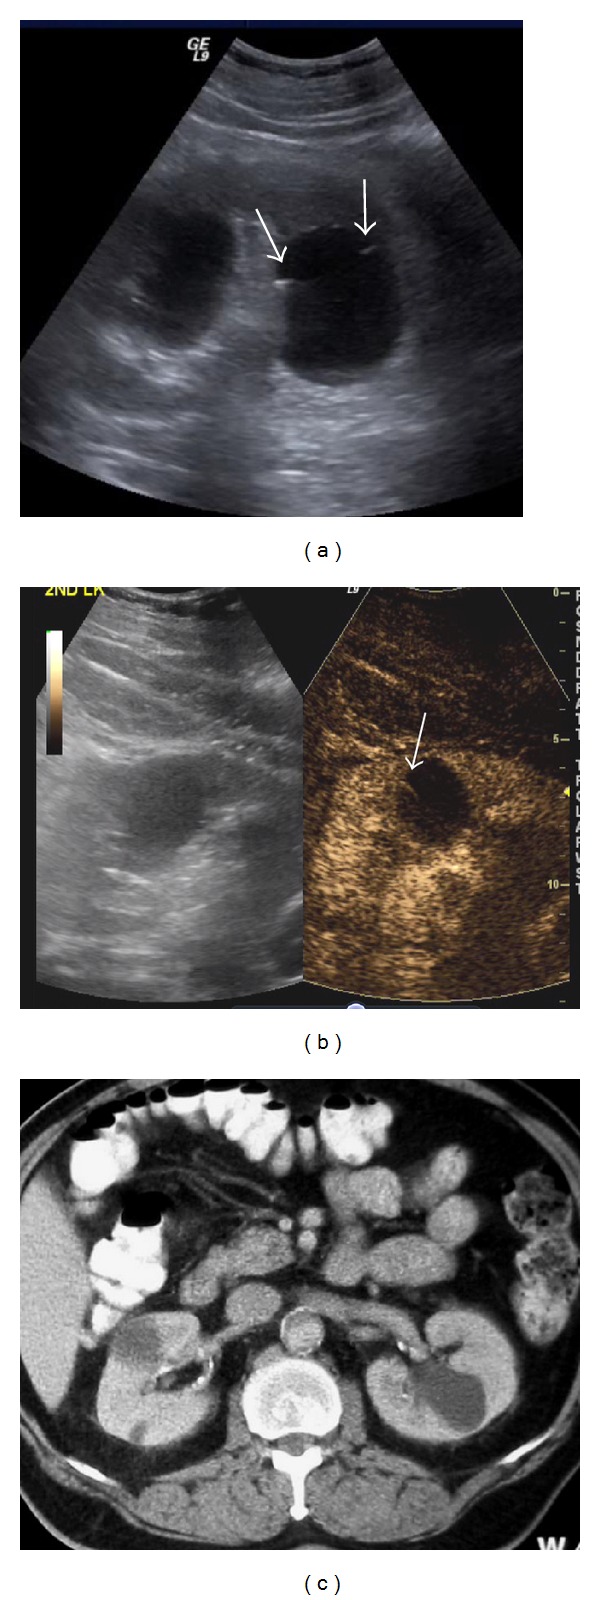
Bosniak IIF cyst: two cysts are present in the left kidney on B-mode US (a). The larger cyst shows small marginal septa (arrows). On CEUS the septa show definitive enhancement (arrow in (b)). This classifies the cyst as IIF. This enhancement is not evident on contrast enhanced CT (c).
